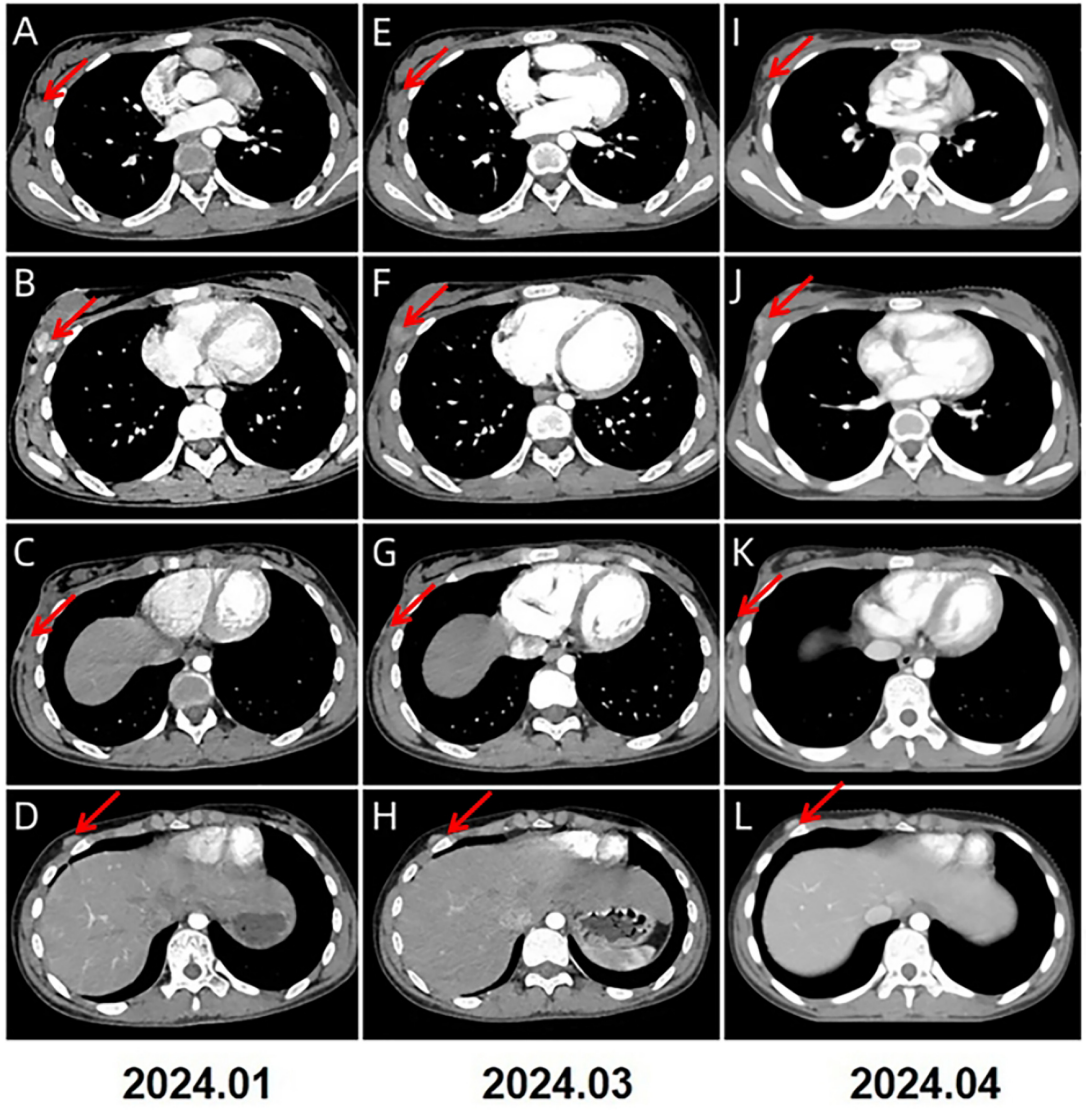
2024.1–2024.4 (A–L) CT imaging showed a significant reduction of the lesions in size, with the largest now measuring 1.2 × 1.1 × 1.0 cm (indicated by the red arrows).
